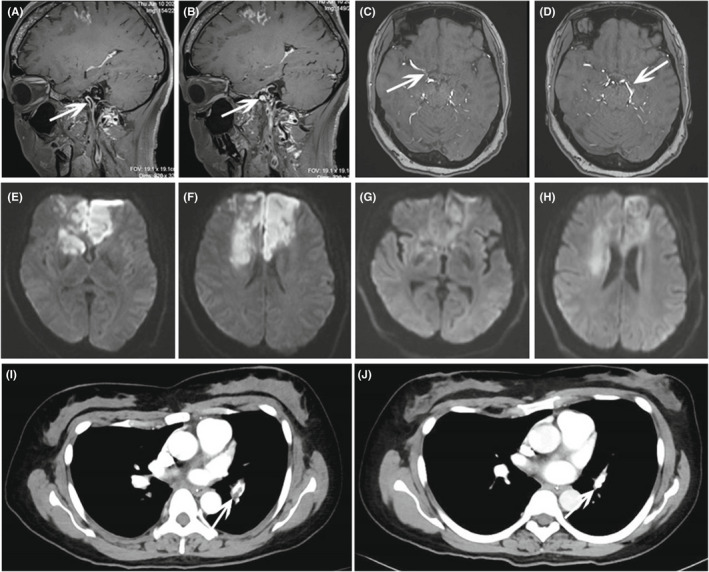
(A, B) Sagittal vw‐MRI showed smooth, homogeneous, concentric arterial wall thickening and enhancement in left ICA. (C, D) Axial MRA revealed the development of fragile collateral network. (E, F) Bilateral frontotemporal lobes and basal ganglia acute ischemic lesions were presented on twelfth day after admission. (G, H) Brain MRI was performed on thirty‐second day after admission and no new lesions appeared. (I) Representative image of pulmonary CTA showed thrombosis in left pulmonary arteries. (J) Pulmonary CTA was repeated before discharge and thrombosis in left pulmonary arteries was vanished
